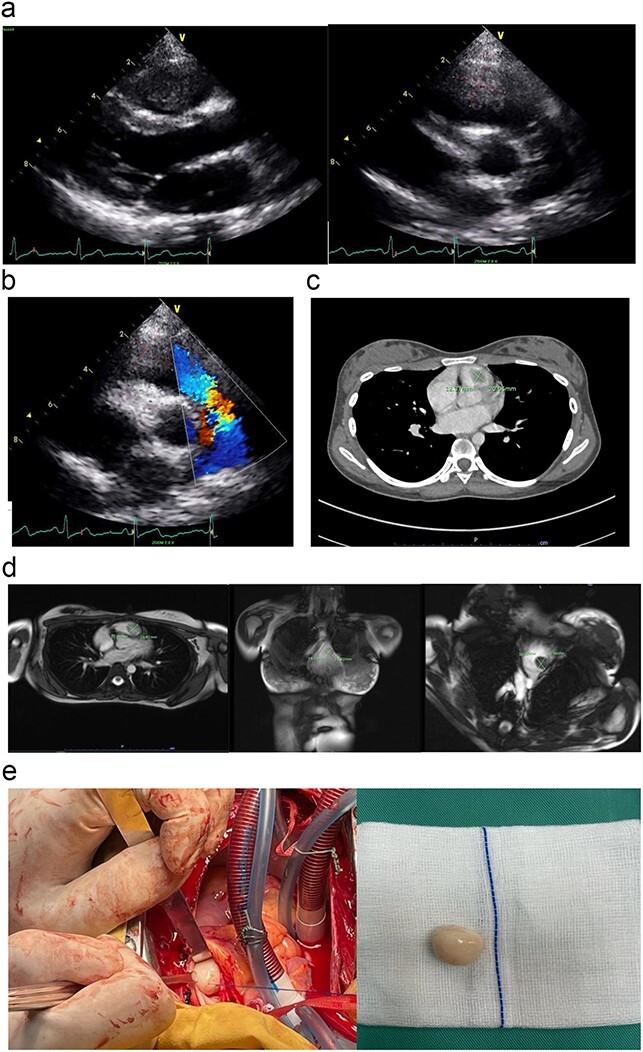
(a) The parasternal long-axis view of transthoracic ECHO showing a well-defined mass, measuring ~ (2.0 × 2.0 cm) at the right ventricle. (b) The transthoracic ECHO colour flow Doppler showed significant pulmonary valve stenosis with potential Right ventricular outflow tract obstruction caused by the intracardiac mass. (c) The axial cut of a contrast-enhanced computed tomography (CECT) thorax showed a well-defined mass measuring ~ (2 cm × 2 cm) inside the RV. (d) The cardiac magnetic resonance imaging (MRI) confirmed the diagnosis of right RV myxoma by showing a well-defined hypointense mass measuring ~ (1.7 × 2.0 cm). (e) The tumour was identified and excised via a median sternotomy, bicaval cannulation, cardiopulmonary bypass, hypothermia, and cardiac arrested via antegrade cold-blood cardioplegia. The tumour was excised via a pulmonary arteriotomy approach. The tumour is well-defined, firm in consistency, and smooth surface. The size is ~ (2 cm × 2 cm).
